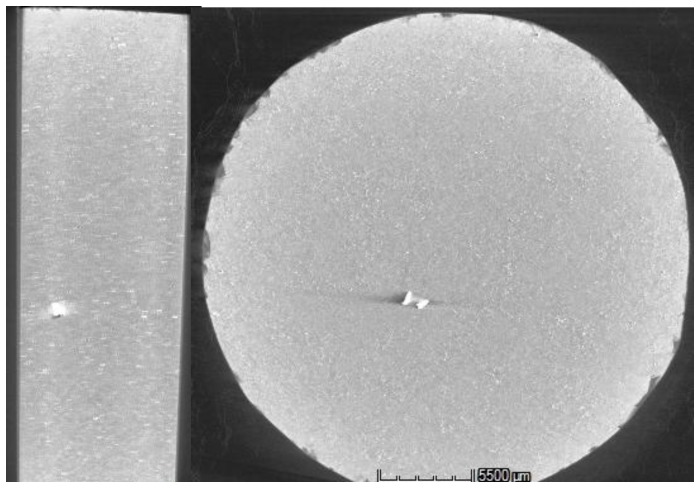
CT images revealing an impurity within the material.
